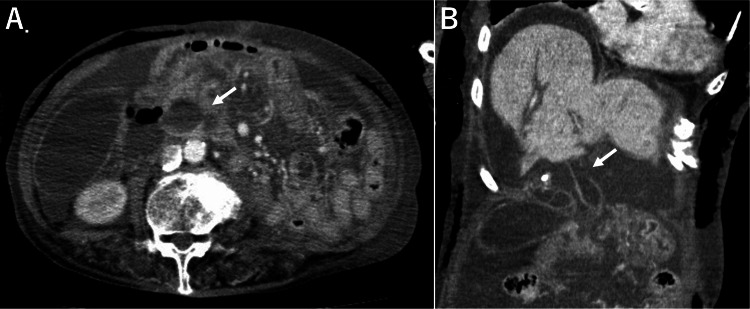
Contrast-enhanced CT on POD 4 showing hepatic duct perforation (A) Dilated common hepatic duct (arrow). (B) A focal contrast defect was observed in the wall of the common hepatic duct (arrow), suggestive of the perforation site. CT: computed tomography; POD: postoperative day
